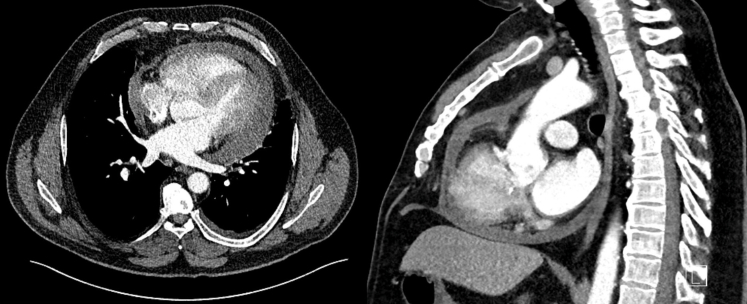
Top:* Transverse plane image of contrast-enhanced computed tomography showing moderate circumferential pericardial effusion measuring 15–30 Hounsfield units. There is a small left-sided pleural effusion also seen. No evidence of contrast extravasation was demonstrated. *Bottom:* A sagittal plan image showing circumferential pericardial effusion, contrast in the left atrium, and no demonstrable communication with the esophagus.
